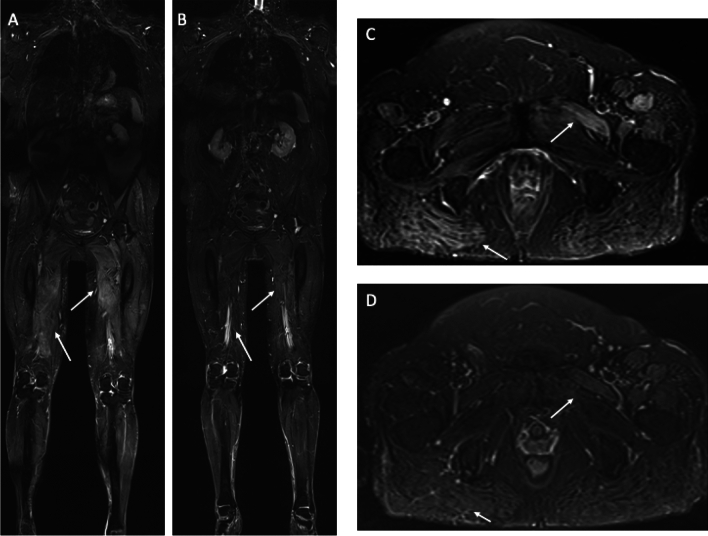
WB-MRI of a 68-year-old woman with necrotizing myopathy. Image in a and c show diffuse muscle edema on the coronal and axial STIR planes. WB-MRI performed as follow-up 2 months later after starting treatment and stopping statin demonstrated a partial resolution of the muscle edema (coronal and axial STIR in b and d). Pathological anatomy requested a search for Anti-HMG-CoA reductase antibodies on suspicion of statin-induced necrotizing autoimmune myopathy (SINAM)
